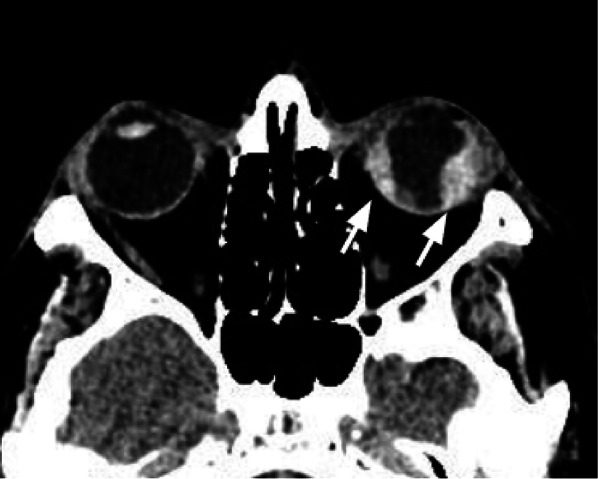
Axial CT acquisition reconstructed with a soft tissue algorithm of a choroidal detachment with choroidal hemorrhage, visible as hyperdense lentiform component on CT (white arrows), that diverges approaching to the optic disc (compared to the retinal detachment that converges to the optic disc)
